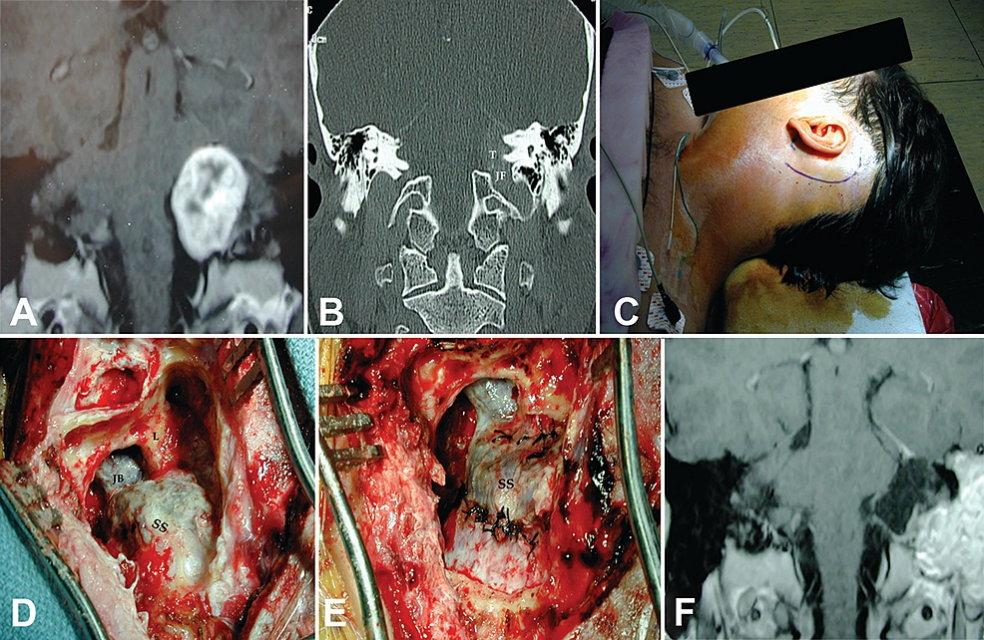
Glossopharyngeal schwannoma accessed via mastoid triangle. Coronal T1 gadolinium-enhanced (A) and computed tomographic scan (B) of a mostly intradural glossopharyngeal schwannoma (case 8). The patient in their late 30s presented with hearing loss, tinnitus, and vertigo, and the tumor was mostly intracranial impinging toward the jugular foramen (type 2). A retroauricular incision was planned (C) and the mastoid triangle was exposed in both its presigmoid and suprajugular sectors (D). A combined presigmoid and retrosigmoid intradural approach was performed (E) to achieve a gross total resection, as seen on the postoperative coronal MRI scan (F). Labels: JB: jugular bulb; L: labyrinth; SS: sigmoid sinus.
